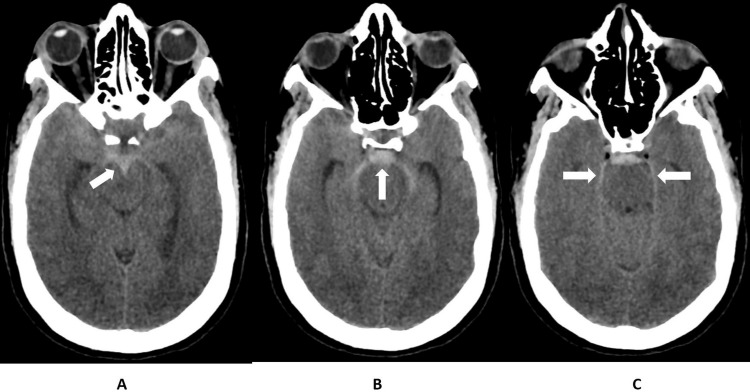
Non-contrast head CT scan showing hyper-densities along basal cisterns with a perimesencephalic hemorrhage pattern (white arrows)
